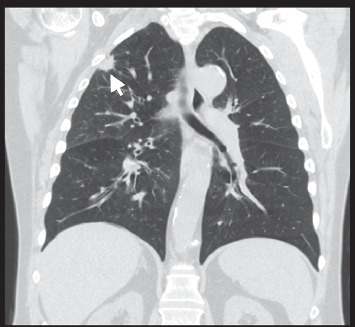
Noncontrast CT chest, coronal view. Visible is a 1.5 cm tumor mass in the right upper lobe of the lung.
